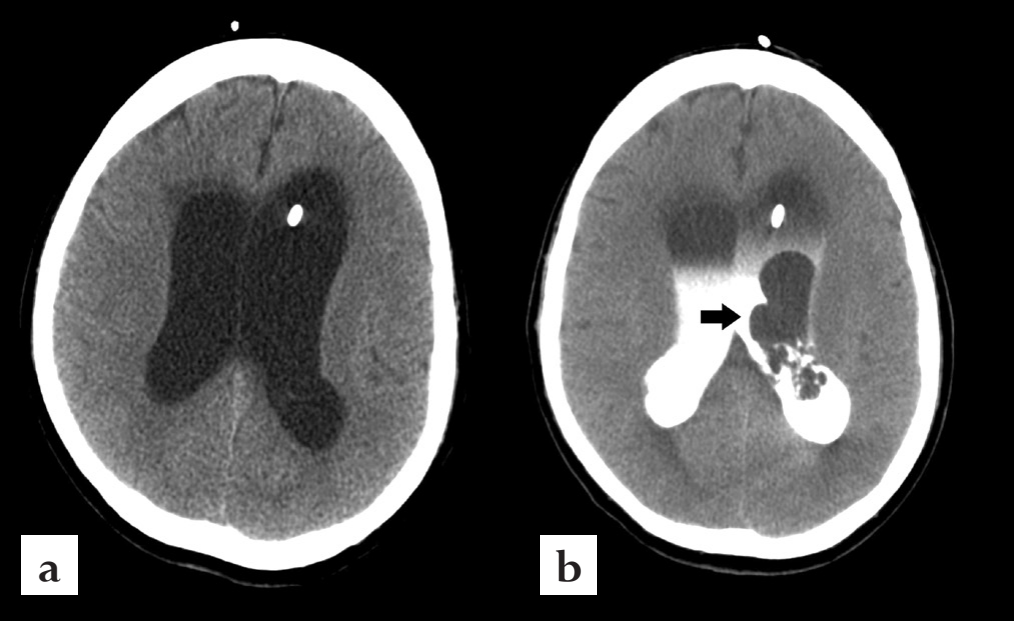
Case #1. (a): CT scan of the brain without contrast showing L frontal ventriculostomy in lateral ventricle. (b): CT scan of the brain during injection of intraventricular iohexol showing multiple lesions in the body of the left lateral ventricle.
